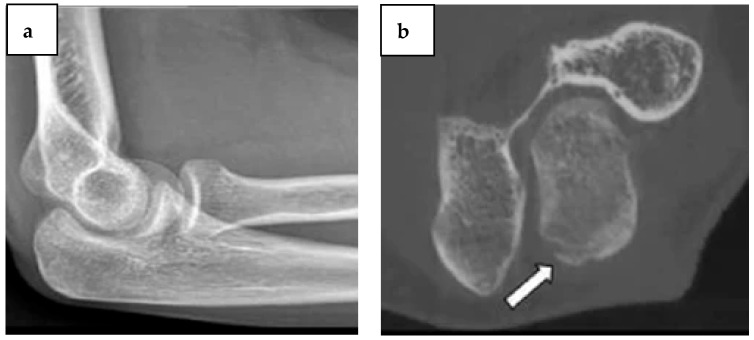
Ulna olecranon occult. (a) The elbow’s lateral view CR reveals no signs of a fracture. (b) An axial CBCT reorganized image at the posterior part of the olecranon process shows an obscure adjacent bone fragment and a subtle disruption of the cortical structure pointed with the white arrow. Published with permission from [16]. Copyright 2015, Springer Nature.
